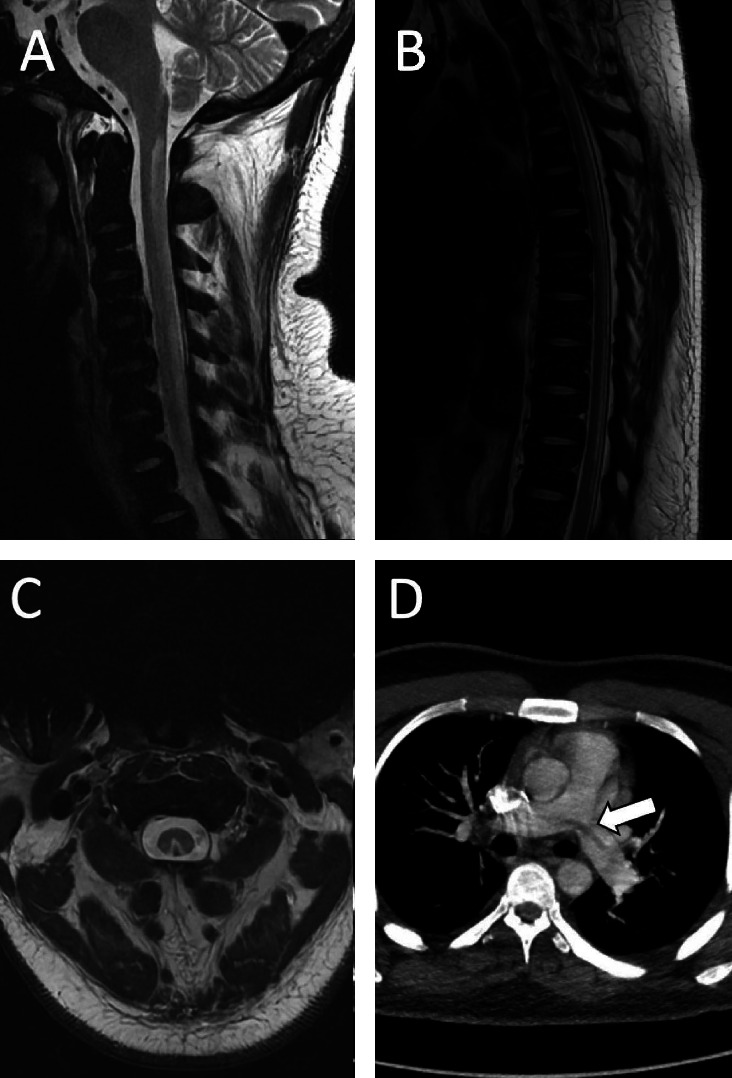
MRI spine showing T2-hyperintensities involving dorsal cervical (A) and thoracic (B) cord with inverted V-sign (C). (D) Saddle pulmonary embolism.
